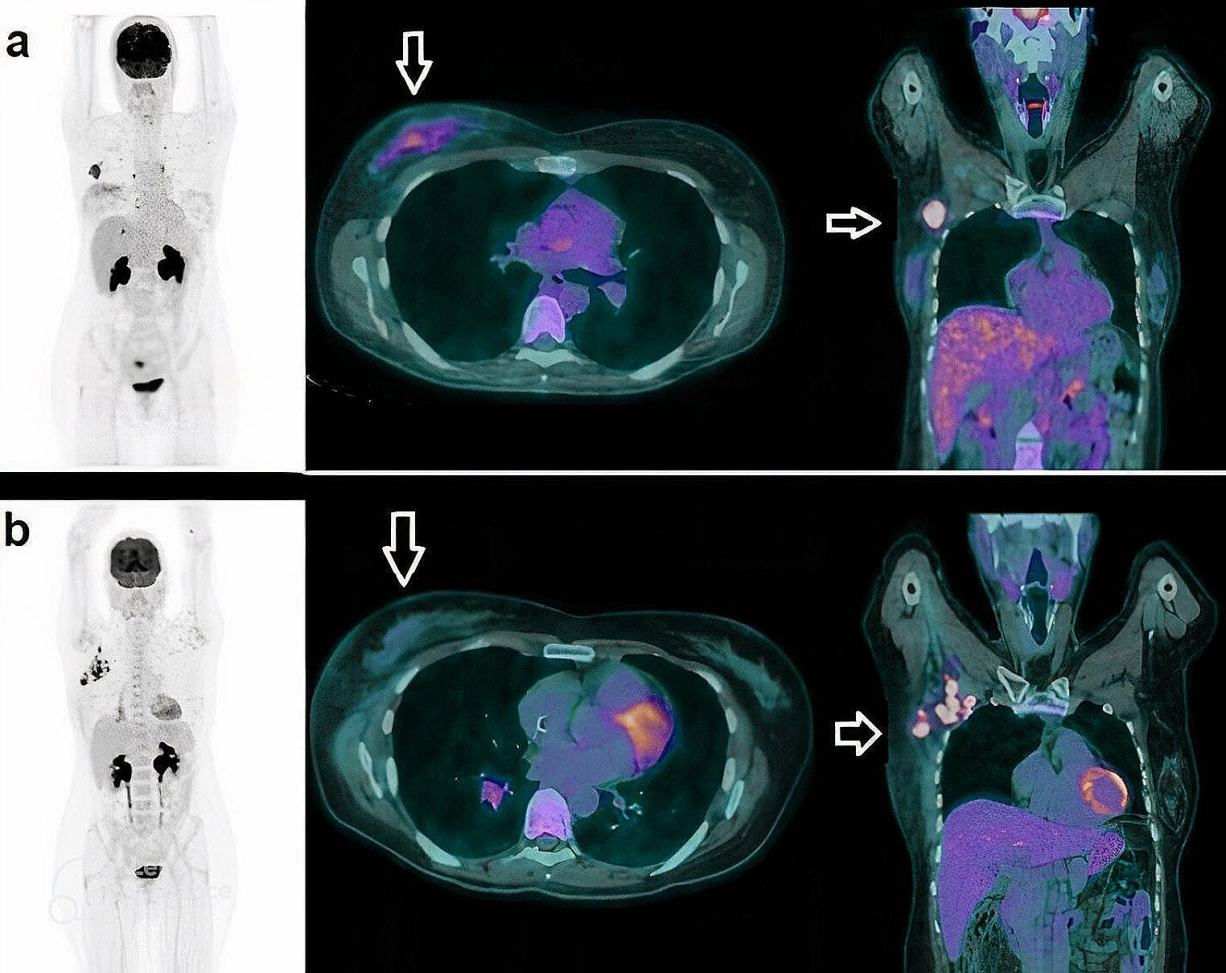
A 42-year-old woman with newly diagnosed triple-negative breast cancer of the right breast. (a) upper row: from left to right, whole body PET, axial and coronal slices before NAC showing highly increased tracer uptake within diffuse alteration in right breast (SUVmax 5, arrow) coupled with an enlarged axillary node (SUVmax 9, arrow); (b) lower row: from left to right, whole body PET, axial and coronal images after 2 cycles of NAC depicting a reduction in tracer uptake in the right breast (SUVmax 2.7, arrow), in spite of an increased number of pathological axillary nodes (SUVmax 12), as for dissociated response. Patient was classified as non-responder and a therapy switch was carried out
